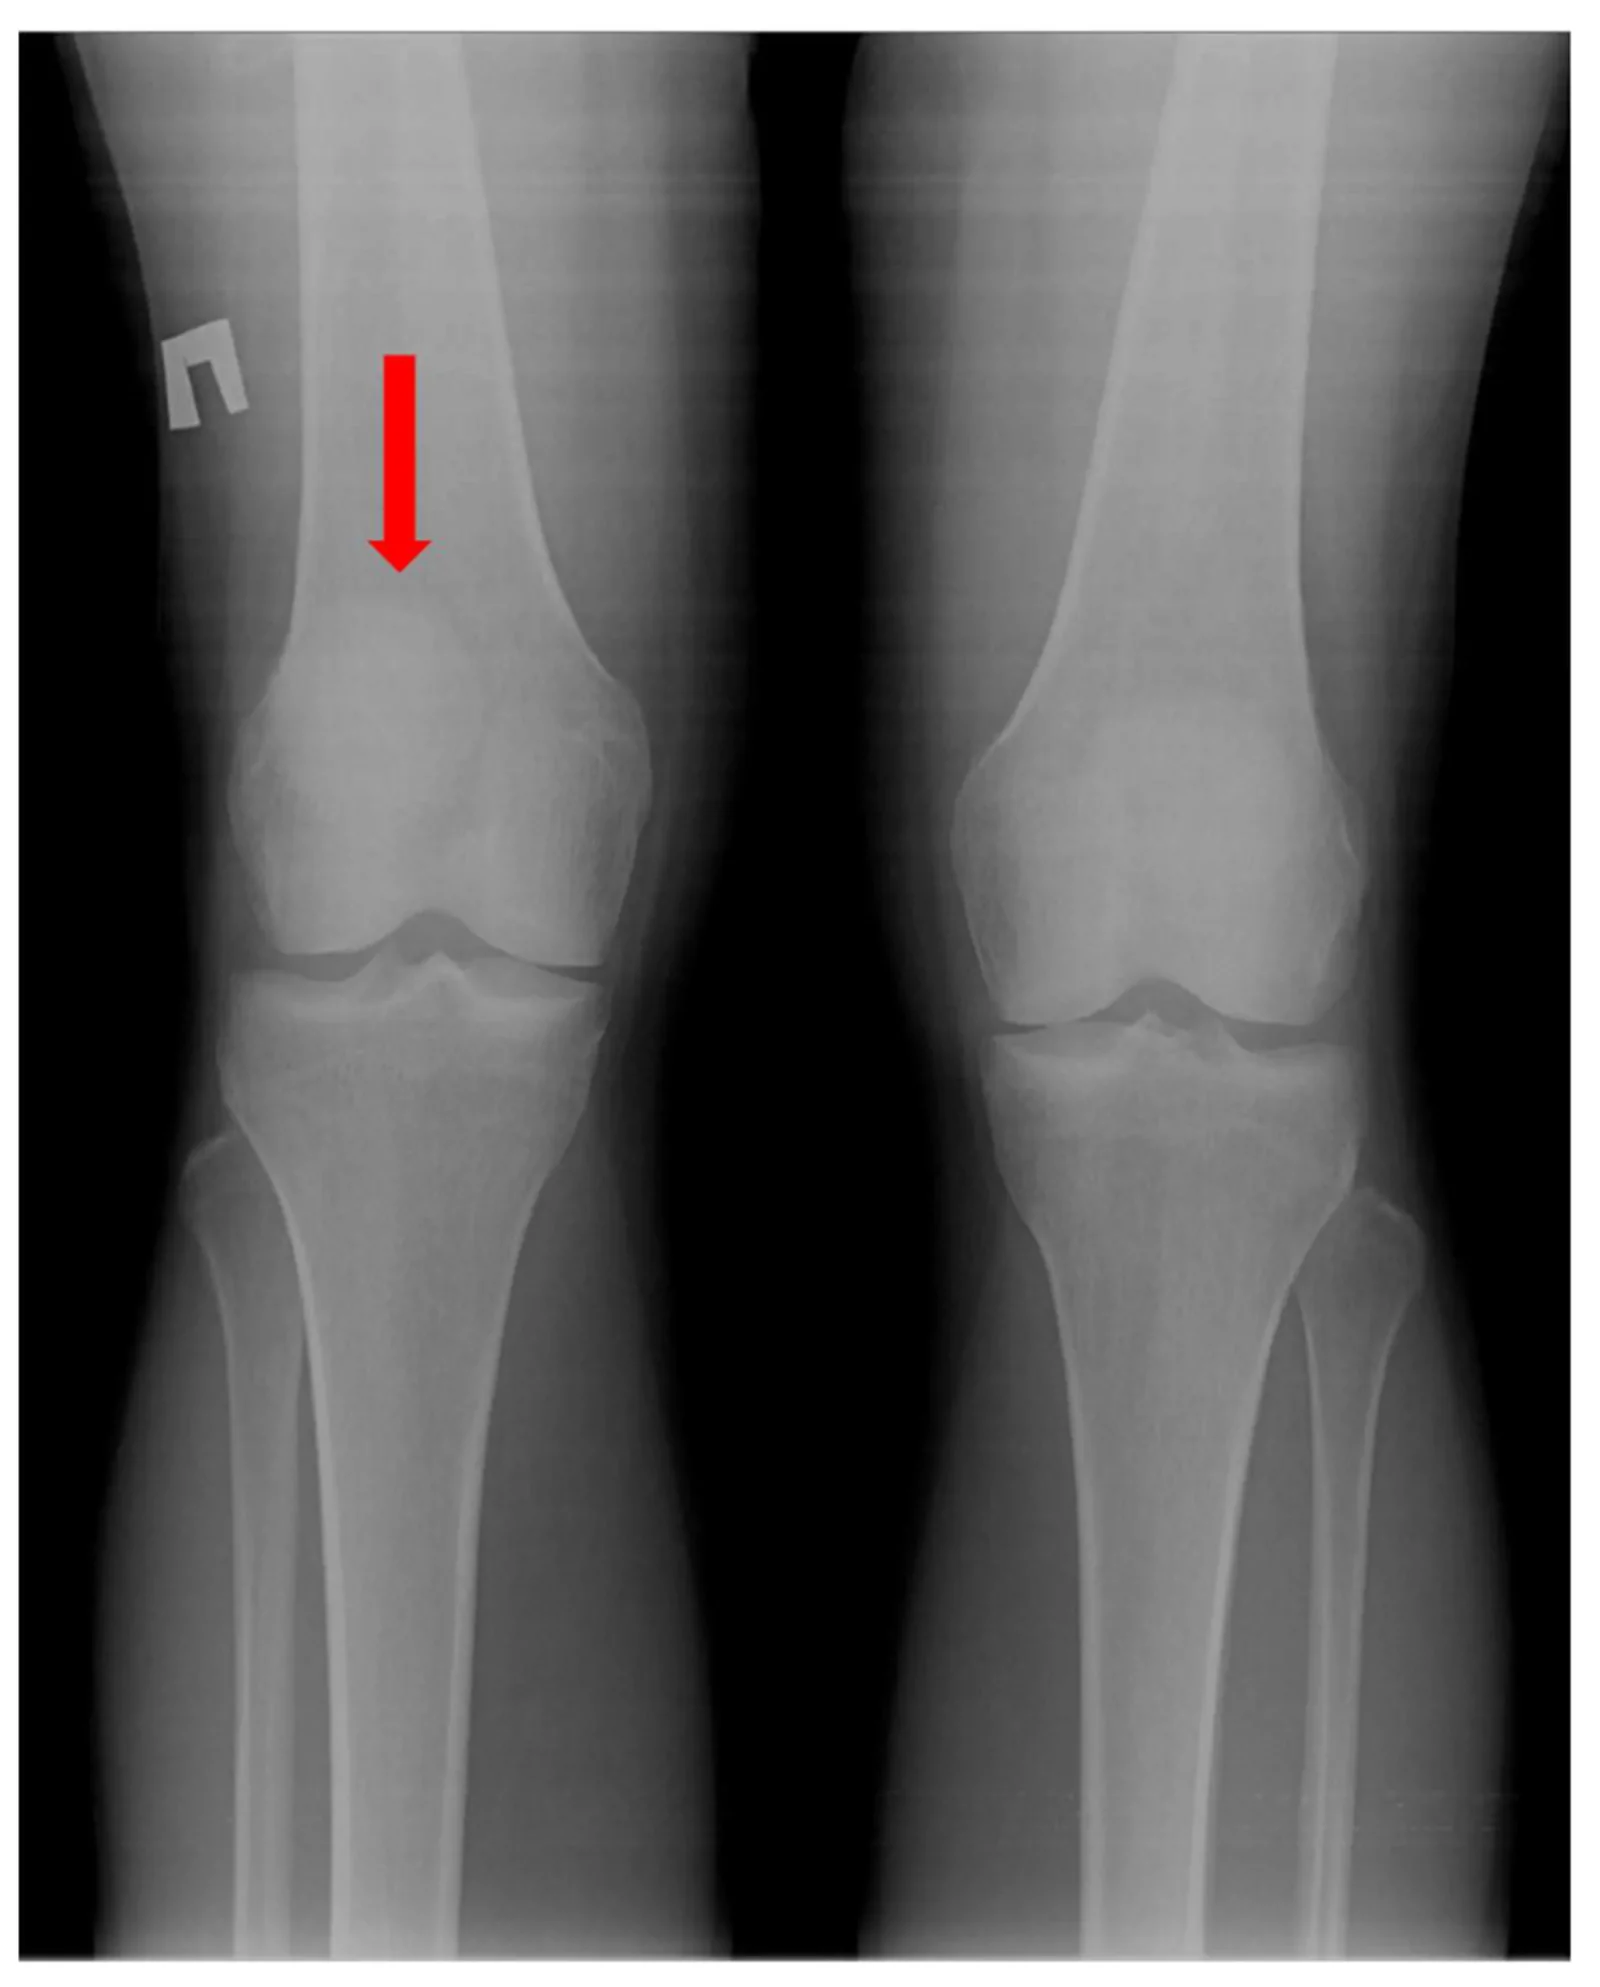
Radiographic imaging of the proband’s knee joints. The knee radiograph demonstrates patella alta on the right side (red arrow).
